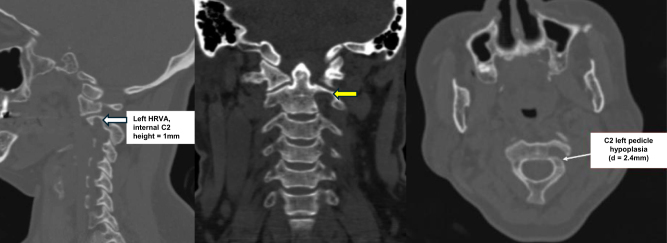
Cervical spine CT scan revealed the narrowing of the left C2 internal isthmus indicating left HRVA, and narrowing of C2 left pedicle.
